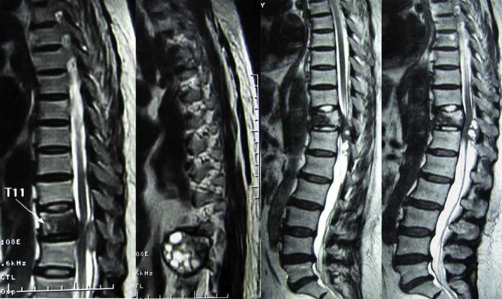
Sagittal T2-weighted MR images showed paraspinal cystic lesion was involved in T11 vertebrae and disc space. Multiple small daughter cysts formed a grape bunch-like image. The thoracic dural sac was narrowed due to the compression of multiple small hydatid cysts like CSF signal intensity.
